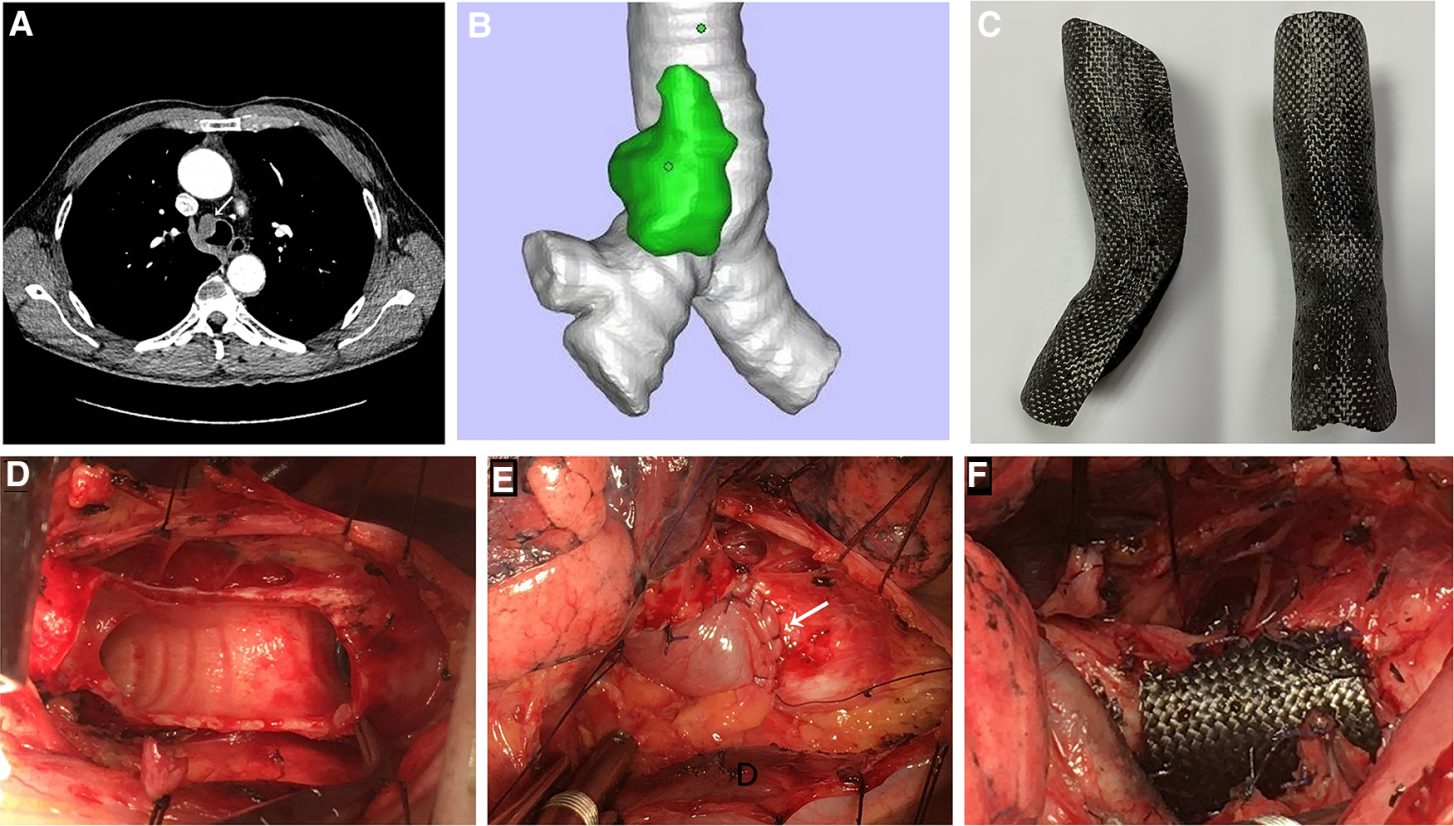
(A) Preoperative CT scan of the thorax. The white arrow indicates a tracheal tumor. (B) 3D model of the trachea with tumor. (C) 3D custom-made carbon fiber stent before tailoring. (D) Trachea defect caused by tumor resection. (E) Defect repaired by using an autologous pericardial patch. The white arrow indicates a pericardial patch. Airway ventilation will cause a patch float at this point. (F) Custom-made carbon fiber stent fixed outside the patch for suspension to abolish a pericardial patch float.
